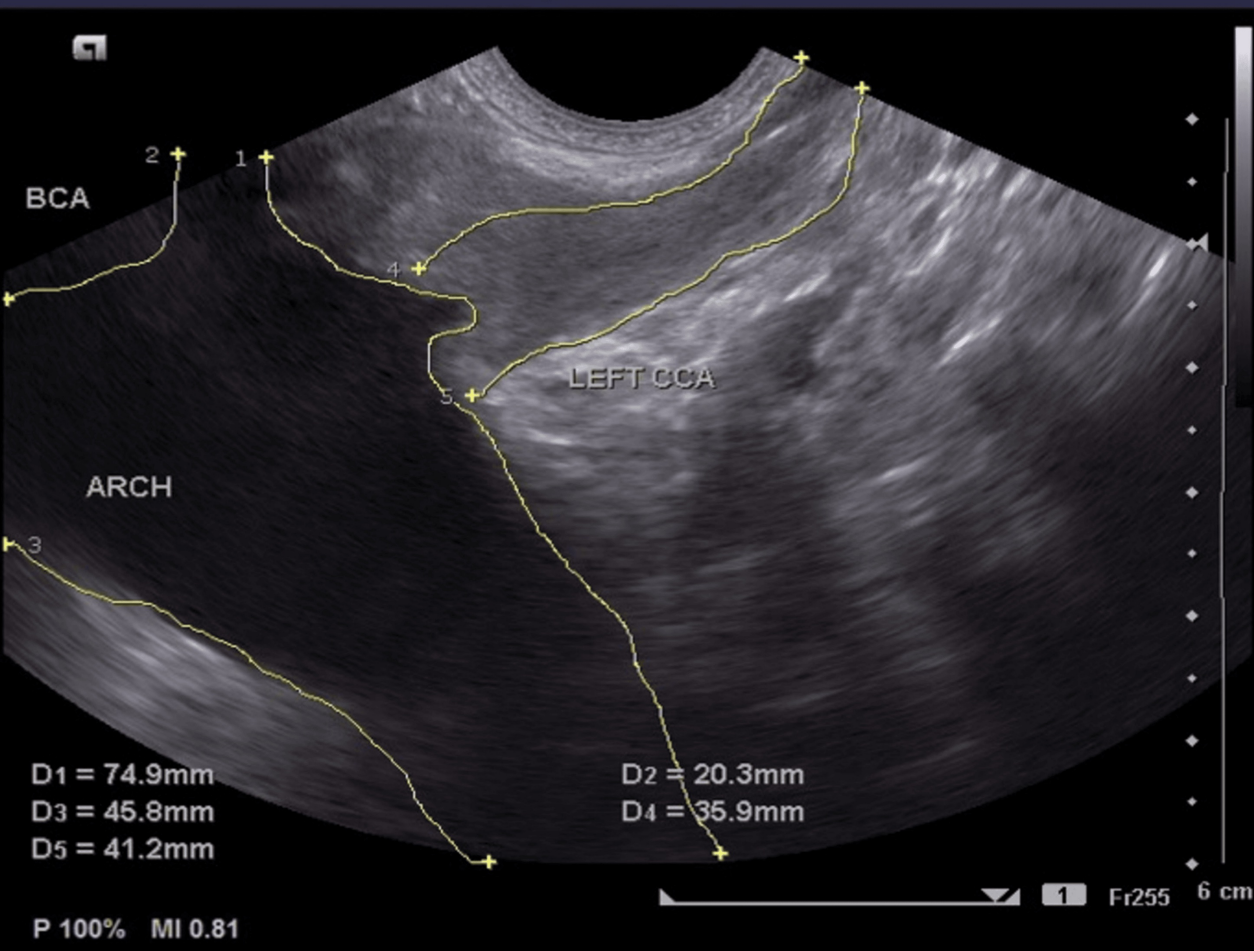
2D sonogram of the aortic arch depicting complete occlusion of the entire left CCA (blue arrow) 2D: two dimensional; BCA: brachiocephalic artery; CCA: common carotid artery
